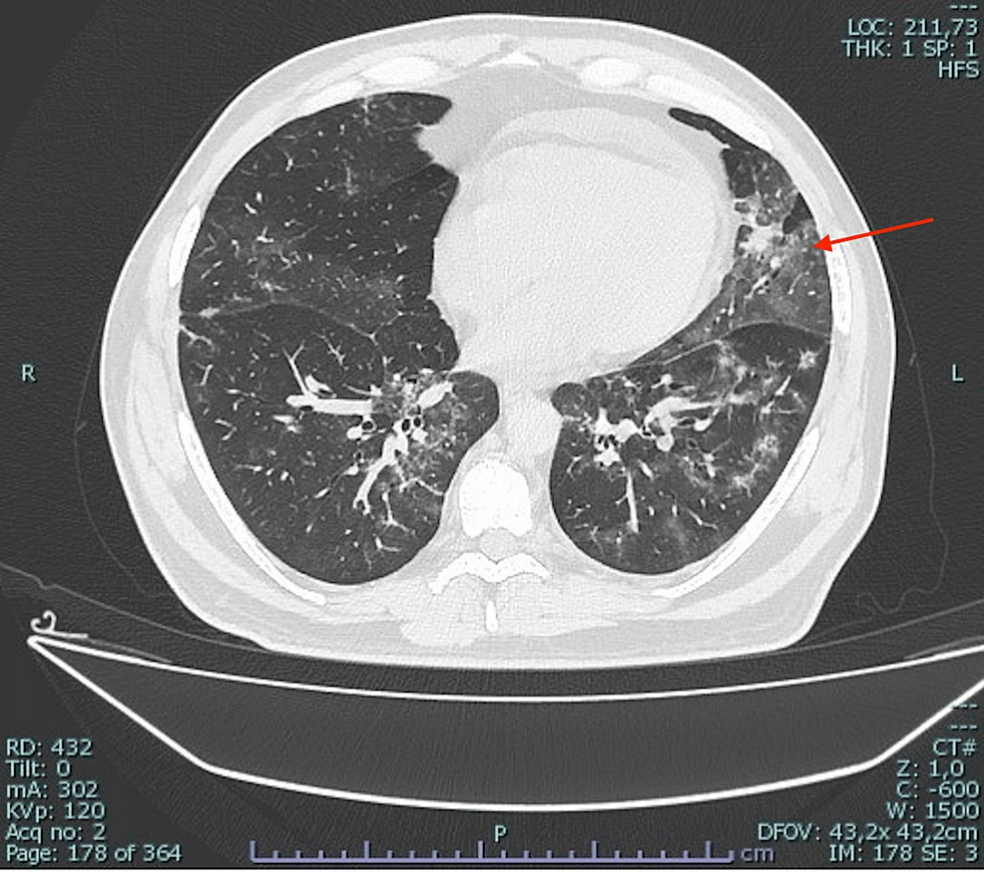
Chest CT shows a bilateral patchy distribution of ground-glass areas associated with consolidation; the arrow indicates a consolidation in the left lung lower lobe.
